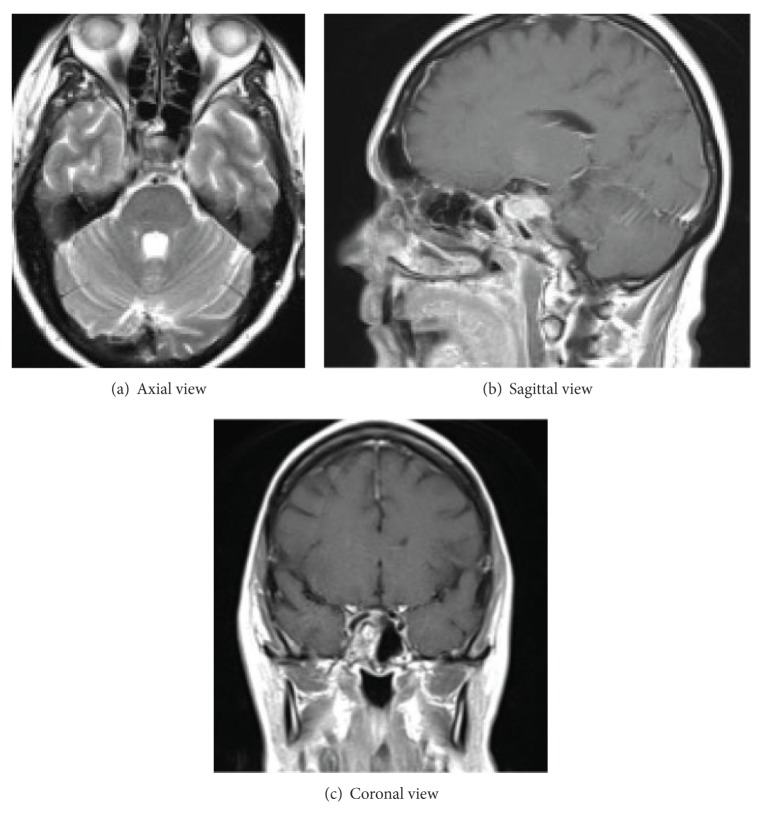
MRI: axial (a), sagittal (b), and coronal (c) views show right cavernous sinus tumor remnant after surgery and radiation.
